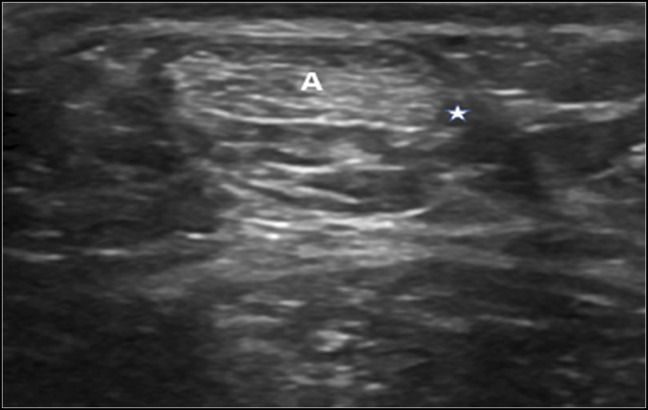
More inferior transverse sonogram demonstrating the anteromedial position of the distal plantaris tendon (*) regarding the Achilles tendon (A).
